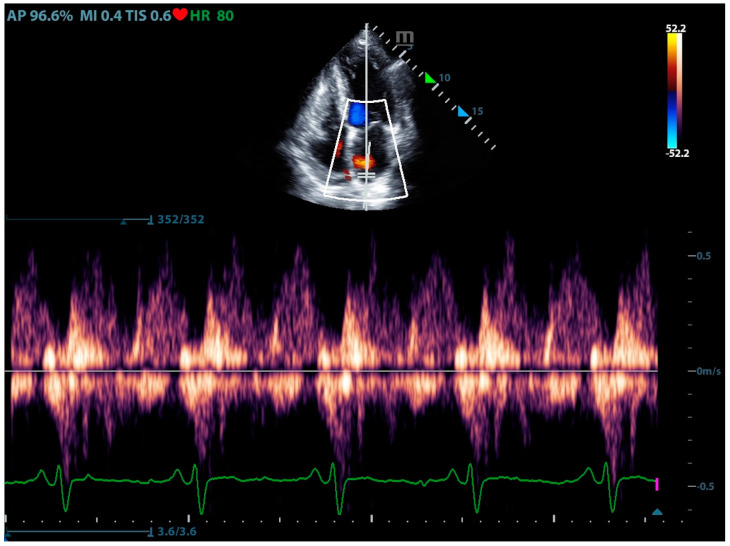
The ultrasound sign of pulmonary venous excess waveform.
